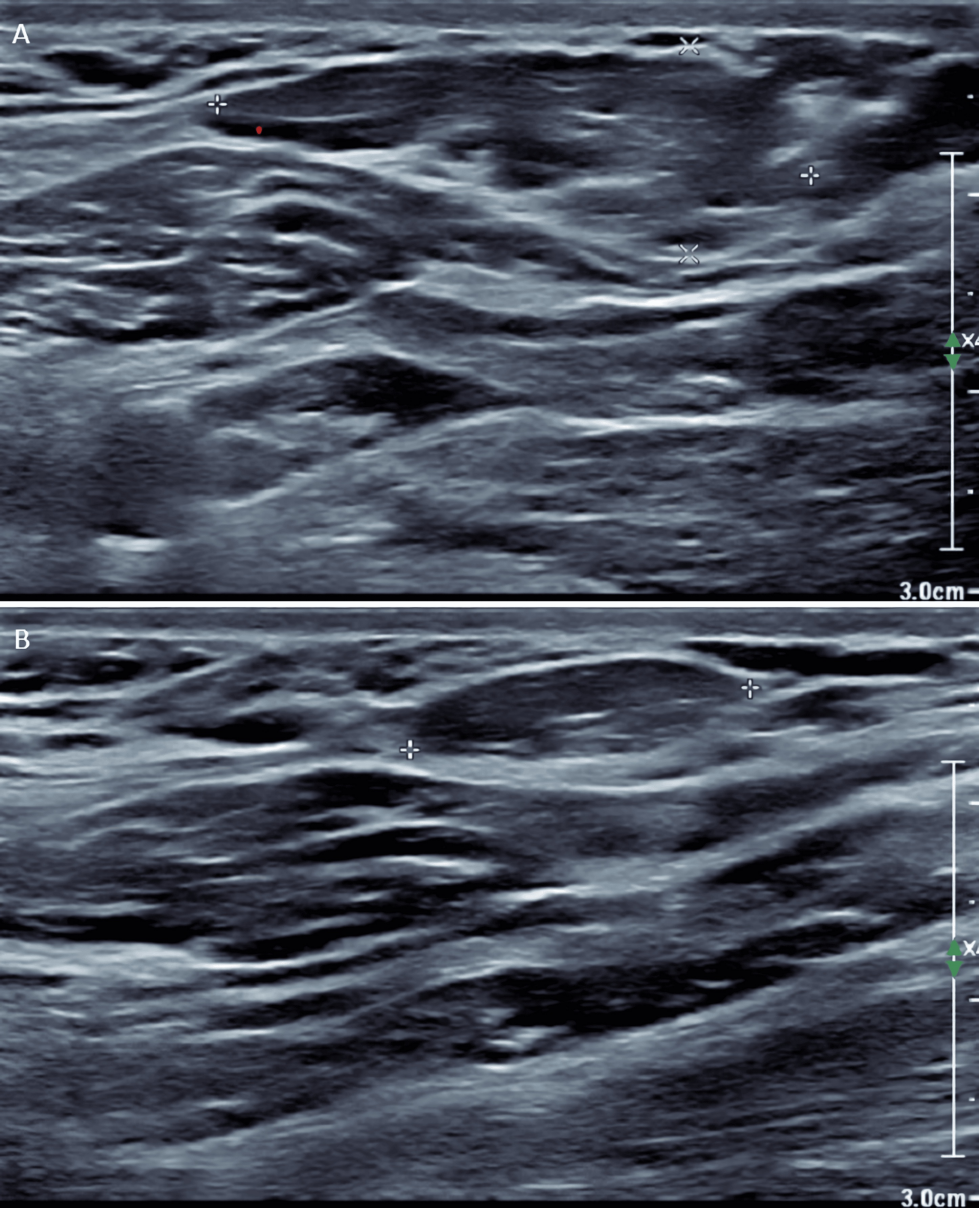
Neck ultrasonography demonstrating sonographic features consistent with a simple lipoma (A) and normal cervical lymph nodes (B).
